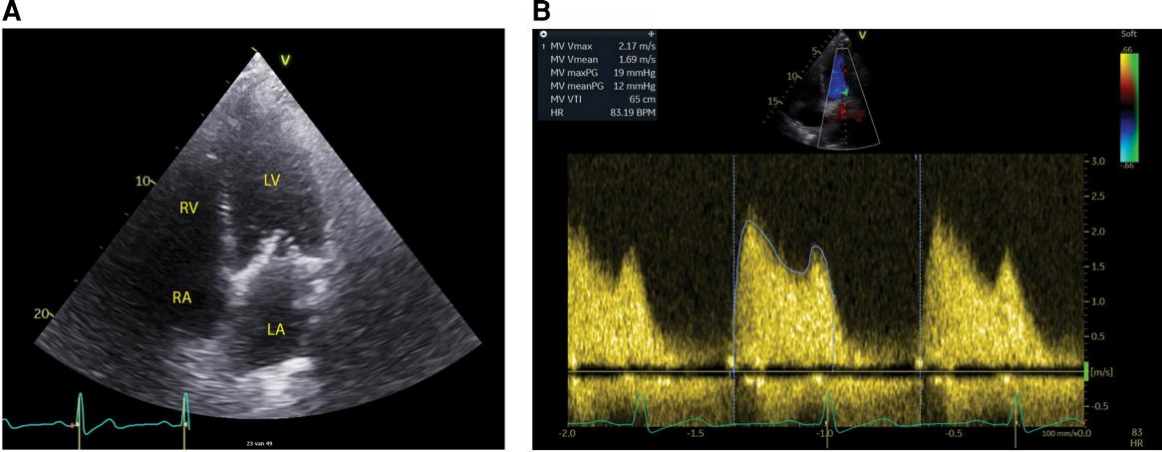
Preoperative transthoracic echocardiography four-chamber view (*A*) showing severely thickened mitral valve cusps and pressure assessment (*B*) of the mitral valve showing a mean pressure gradient of 12 mmHg and a maximum pressure gradient of 19 mmHg. PG, pressure gradient; LA, left atrium; LV, left ventricle; RA, right atrium; RV, right ventricle.
